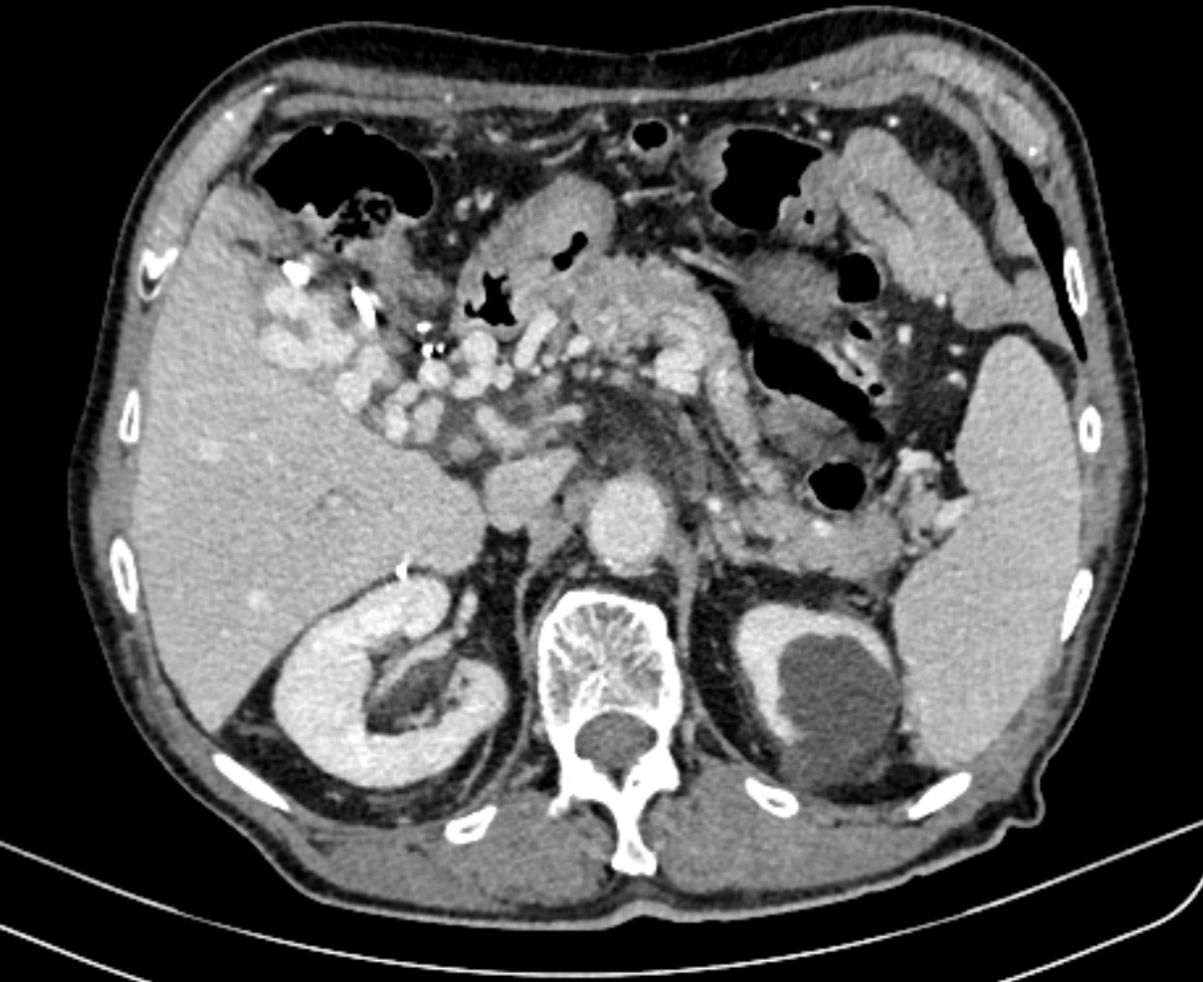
CT scan showing an expansive process of caudate lobe 5.4x3.7 cm in diameter next to portal vein thrombosis and a fatty infiltration of the rest of the liver parenchyma.
